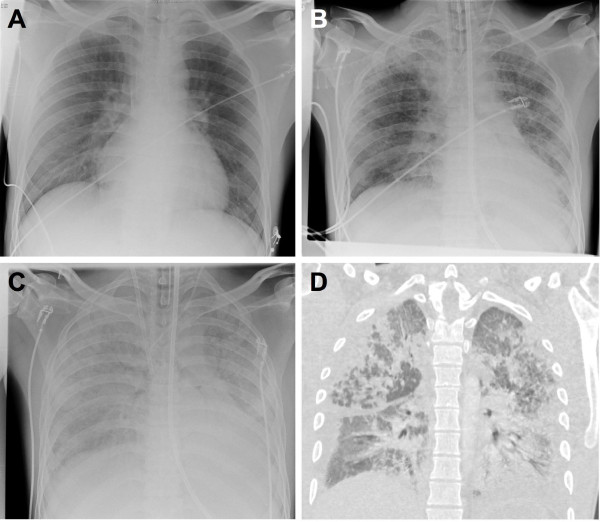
Progressive pulmonary fibrosis. Chest X-ray (A-C) and computed tomography (D; 11th day) demonstrating progressive pulmonary fibrosis on day 3 (A), 9 (B), and 12 (C).
